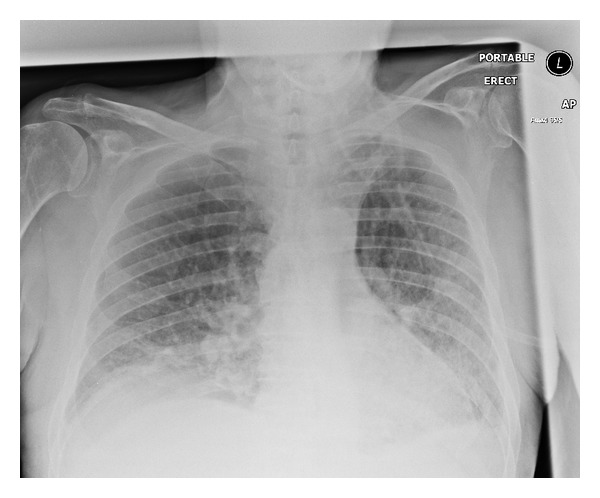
Chest X-ray on day two of admission.
